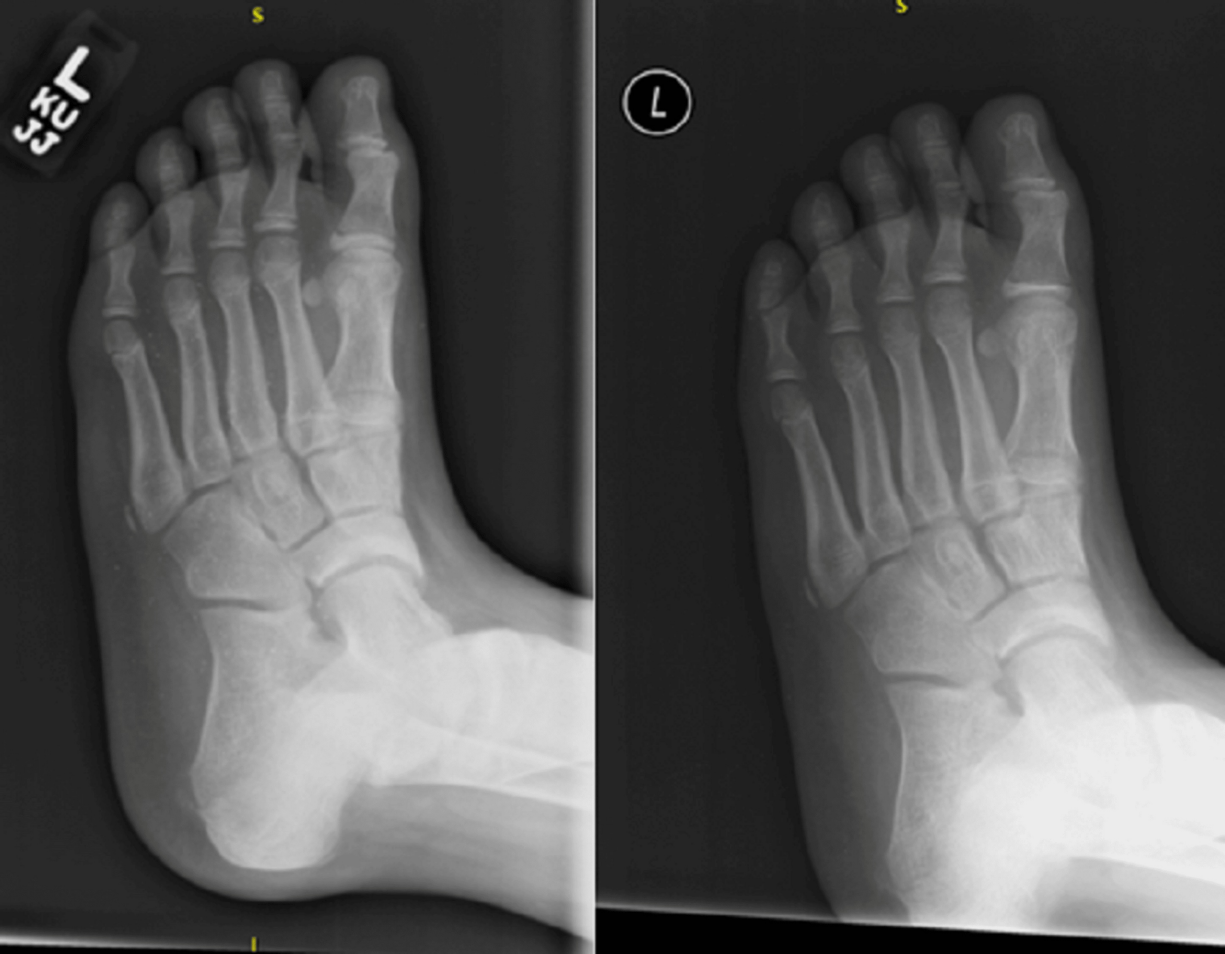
Outside hospital left ankle radiographs at one-month status post injury (A) and two-month status post injury (B) without obvious abnormalities.
